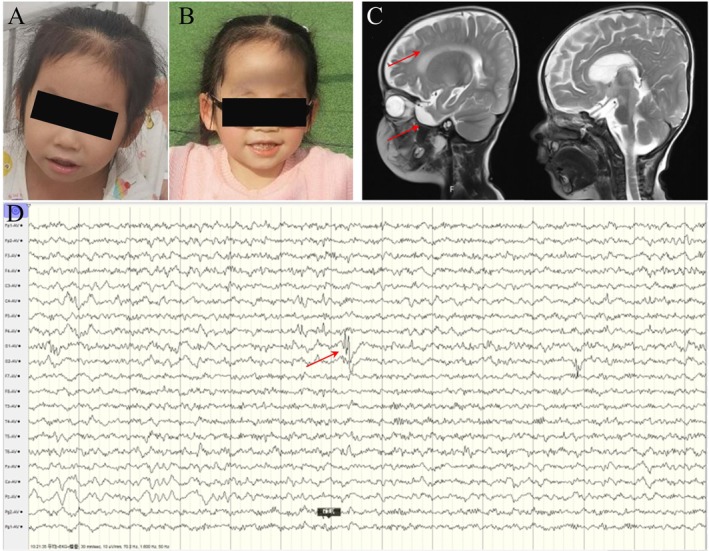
(A, B) Clinical photographs of the patient. (C) Sagittal MR images of the head; red arrows denote abnormal anatomical structures. (D) EEG tracing; the red arrow indicates an episode of abnormal electrical discharge.
